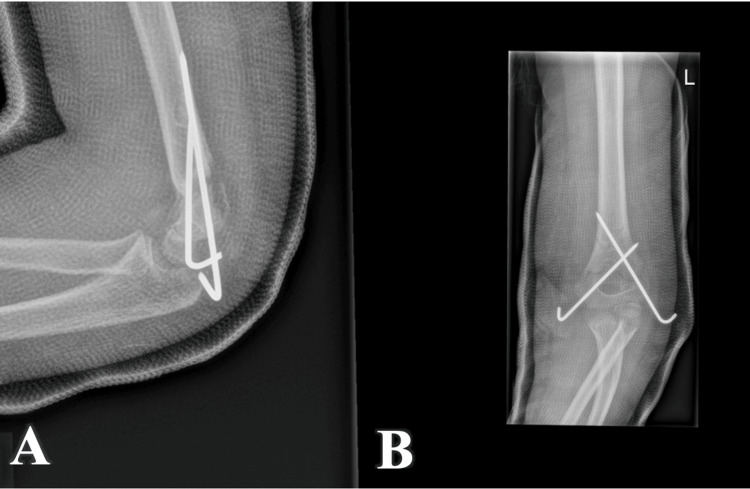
Follow-up radiograph one week after the procedure. A - Lateral view of the elbow, with K-wires in situ; B - Anteroposterior view of the elbow, with K-wires in situ.
